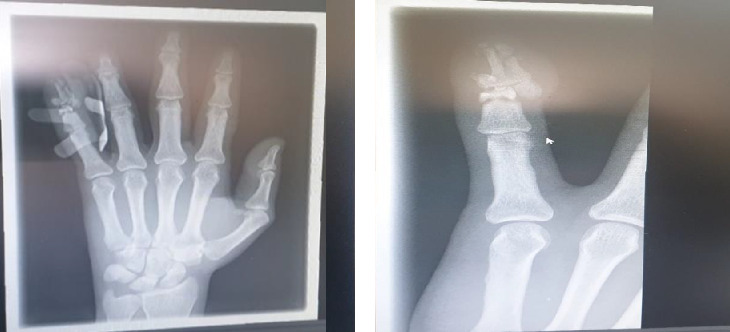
X-ray of (a) the left hand and (b) the left little finger showed that there is a comminuted fracture involving the middle and distal phalanx of the left little finger.
